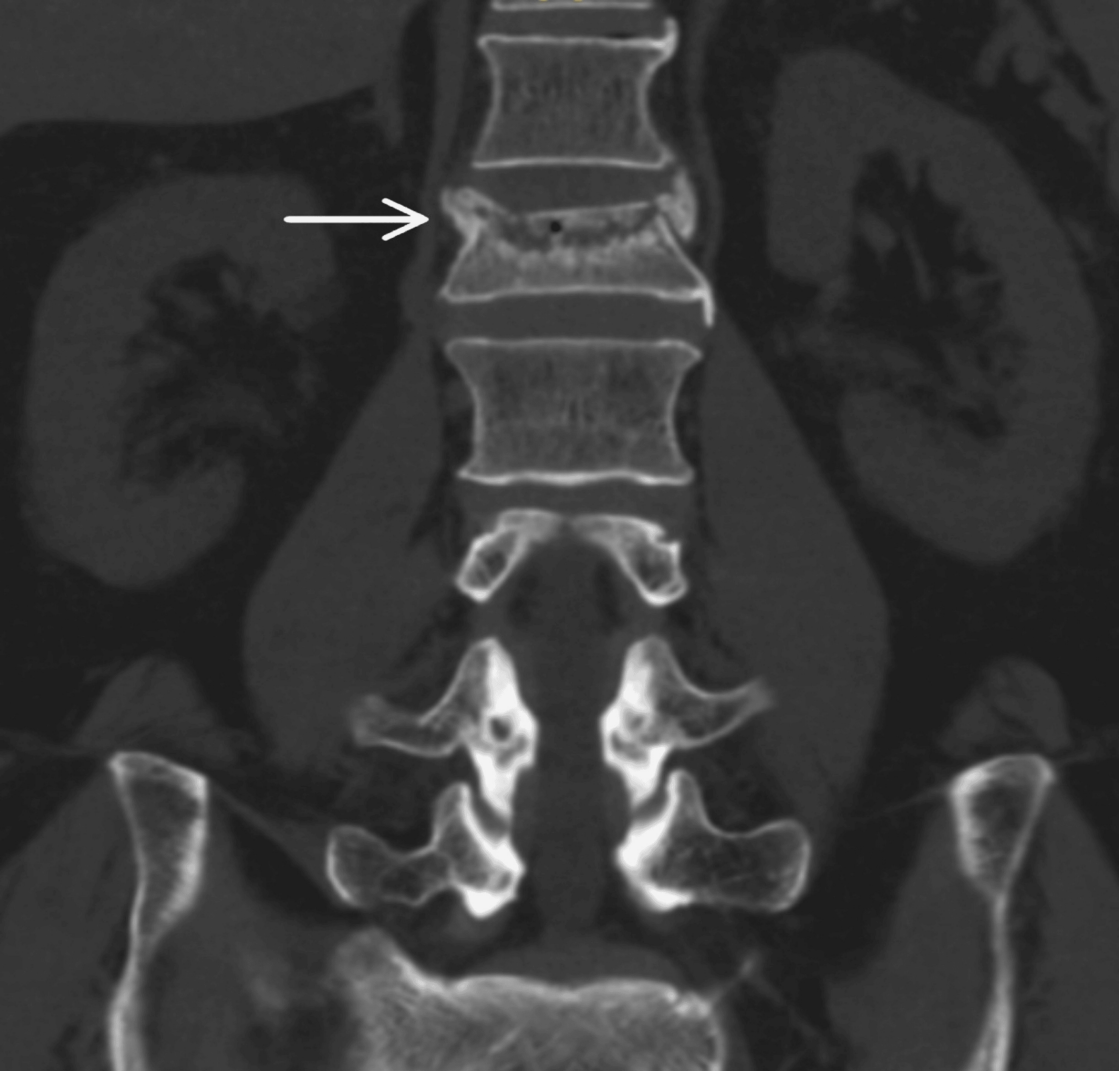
Anteroposterior CT scan view showing L1 vertebral fracture.
